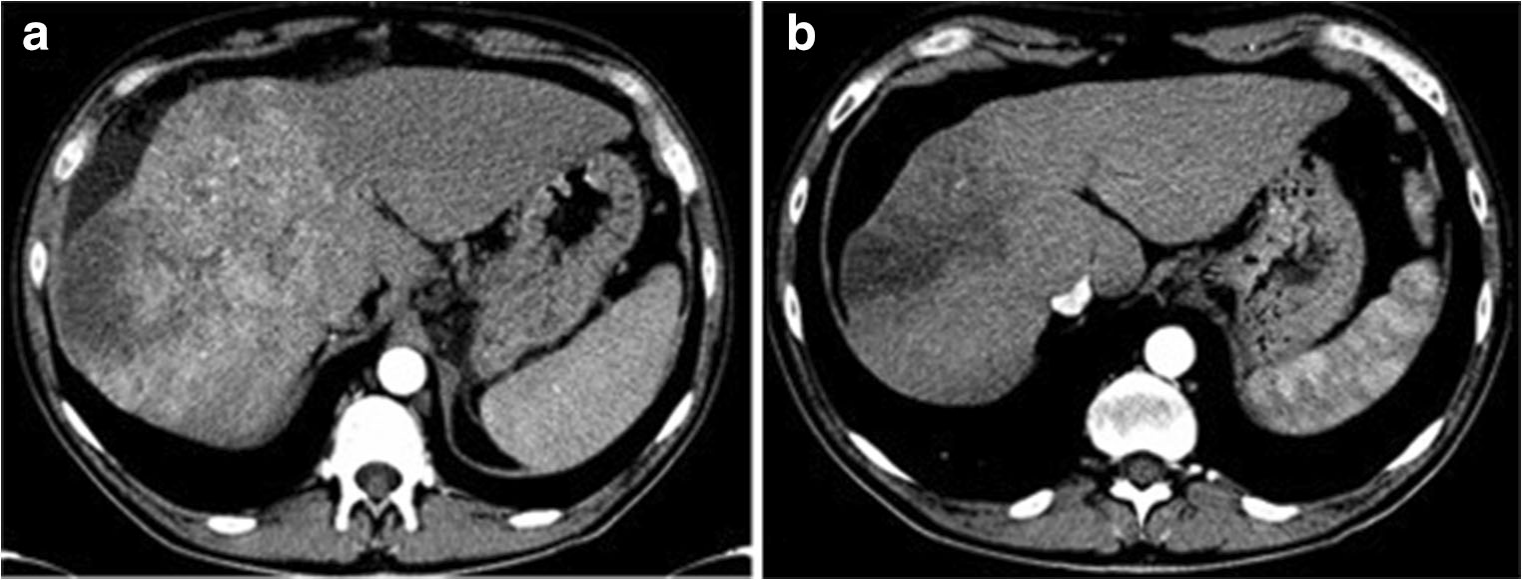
Representative images from a 51-year-old man with advanced HCC and partial response to sorafenib. a Arterial phase spiral CT scan at T0 time shows multiple merging nodular lesions in the right hepatic lobe with a marked enhancement in the arterial phase. b CT scan during arterial phase at T1 time shows a reduction in the size of the right hepatic lobe lesions, with significant lessening of vascularisation
